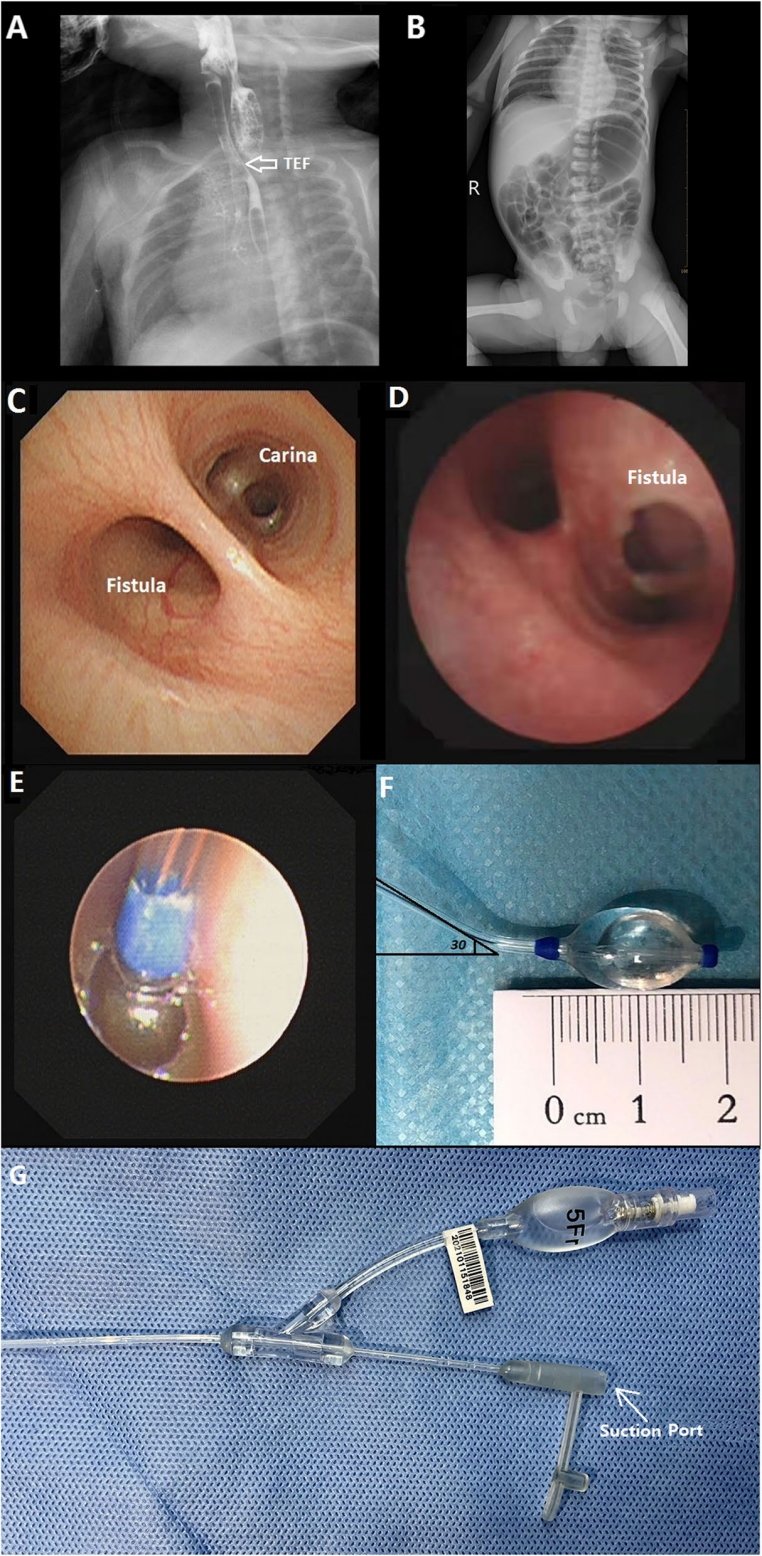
A Preoperative upper gastrointestinal contrast imaging. The white arrow indicates the opening between the esophagus and the trachea. B Posteroanterior chest radiograph shows severe gastrointestinal distension and bilateral pneumonia. C Bronchoscopic view of a TEF. The fistula opening is located 1 cm above the carina. D The opening of the fistula is located at the carina. E Under bronchoscopy, the balloon of the BTBB is inflated with normal saline, and the blue marker is clearly shown. F The size and angle of the 5-Fr BTBB are shown. G The suction port and pilot balloon of a 5-Fr BTBB are shown. TEF = tracheoesophageal fistula; BTBB = balloon-tipped bronchial blocker
